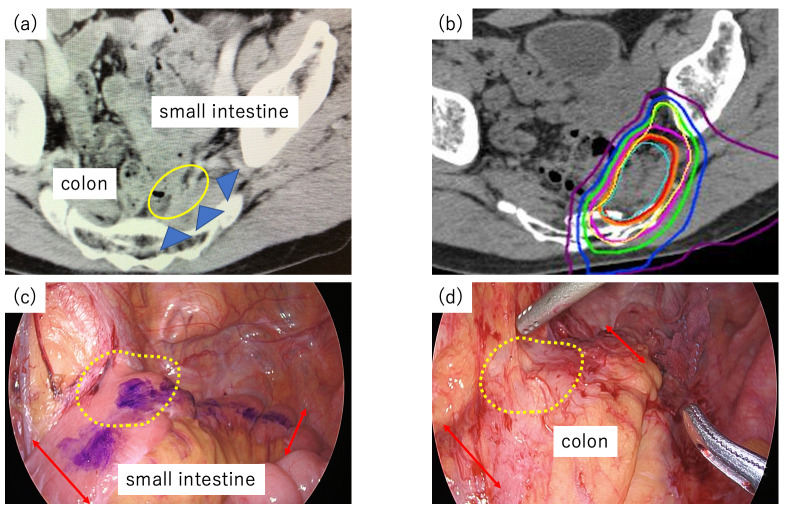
(a) Computed tomography scans showing locally recurrent tumors (yellow circles and blue arrowheads). (b) Depth-dose distribution of carbon-ion beams in recurrent rectal cancer patients. Areas encircled with orange, magenta, green, dark-marine, and violet lines indicate more than 90%, 80%, 50%, 30%, and 10% energy accumulation, respectively. Yellow lines indicate areas of interest for irradiation. (c) The extent of small intestine resection. Surgical resection of the irradiated small intestine with ≥46 Gy (RBE) was planned. (d) The extent of neorectum resection. Surgical resection of the irradiated neorectum of ≥46 Gy (RBE) was planned.
